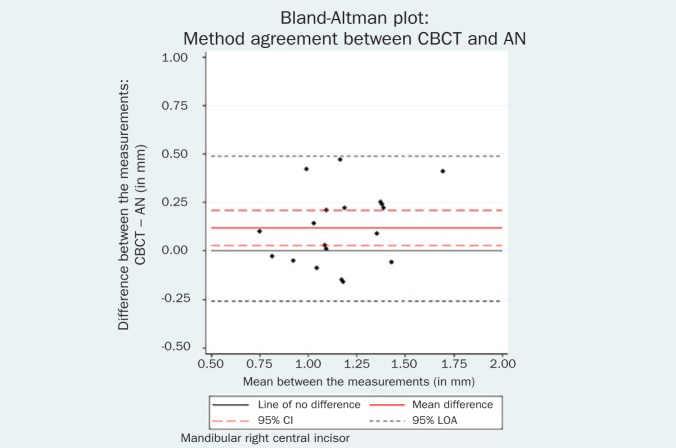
Bland-Altman plot CBCT vs AN 41.
